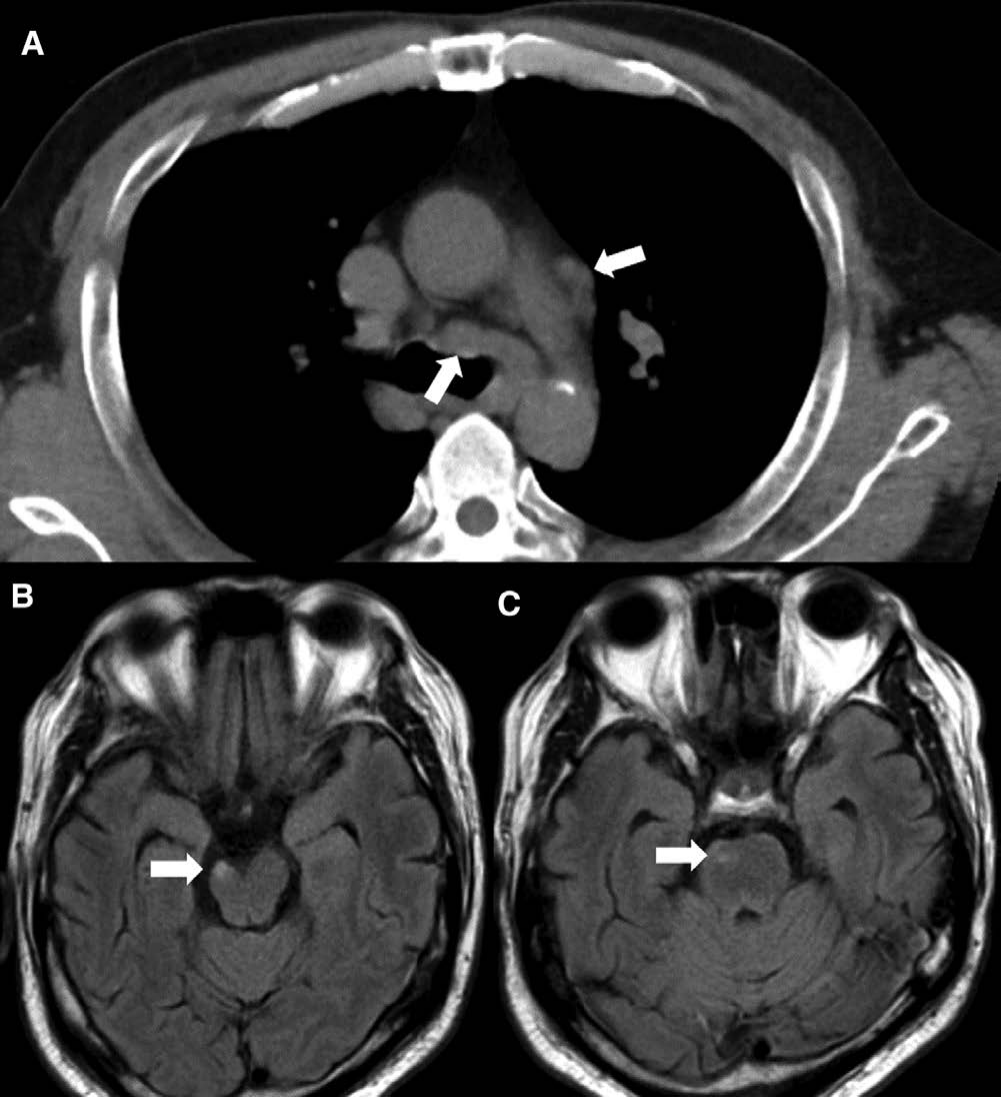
(A) Chest computed tomography. Lymph nodes in the mediastinum are swollen (arrow). (B and C) Head magnetic resonance imaging fluid attenuated inversion recovery images. Although nonspecific as neurosarcoidosis, high-signal areas are founded in the right ventral side of cerebral peduncle (B: arrow) and the pons (C: arrow).
